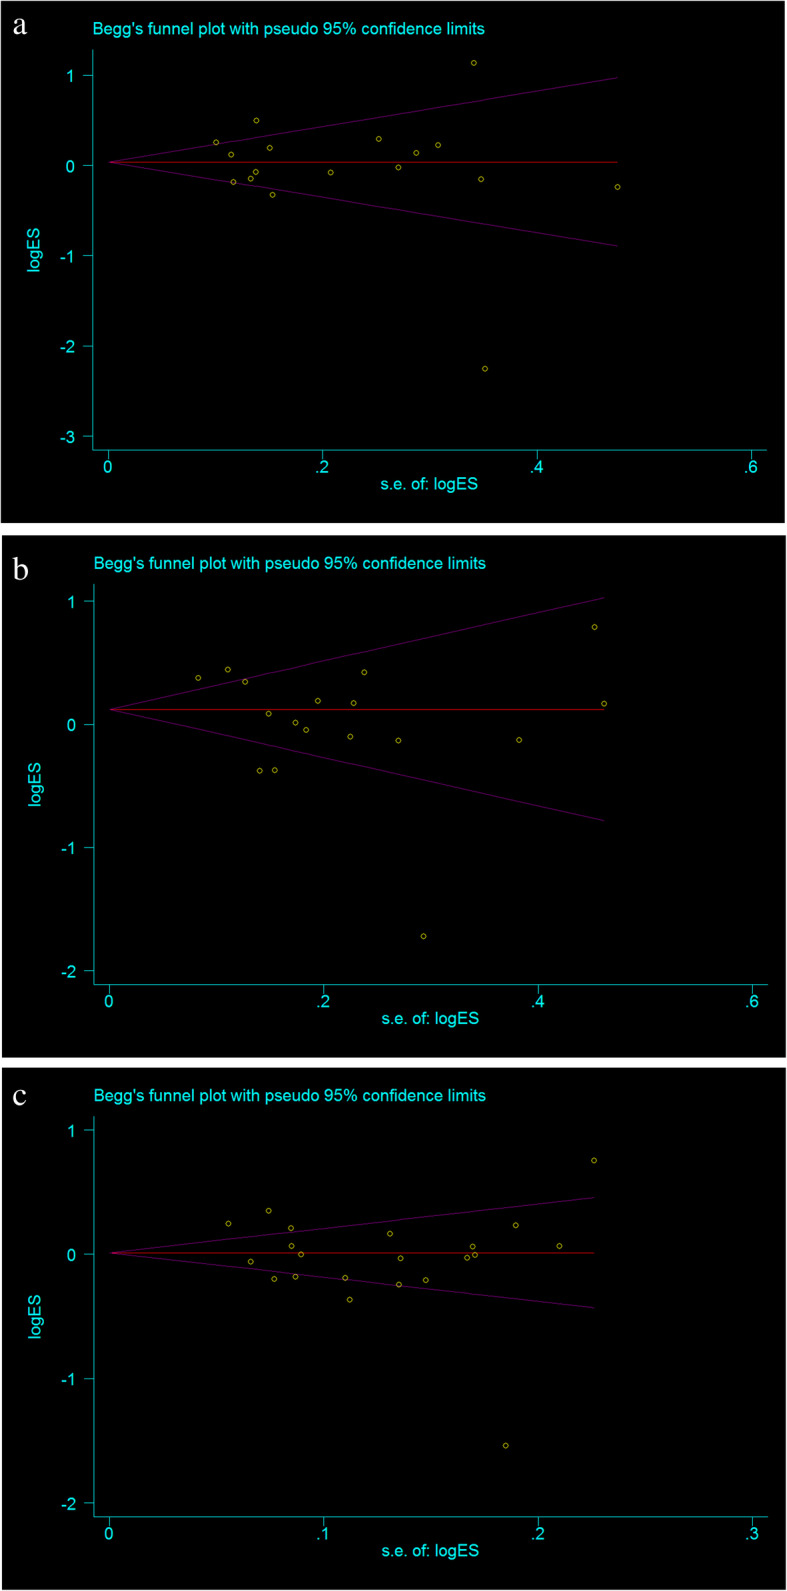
Sensitivity analysis examining the association between the *CEBPE* rs2239633 polymorphism and risk of childhood ALL under these models (CC vs CT + TT, CC + CT vs TT, C vs T)
